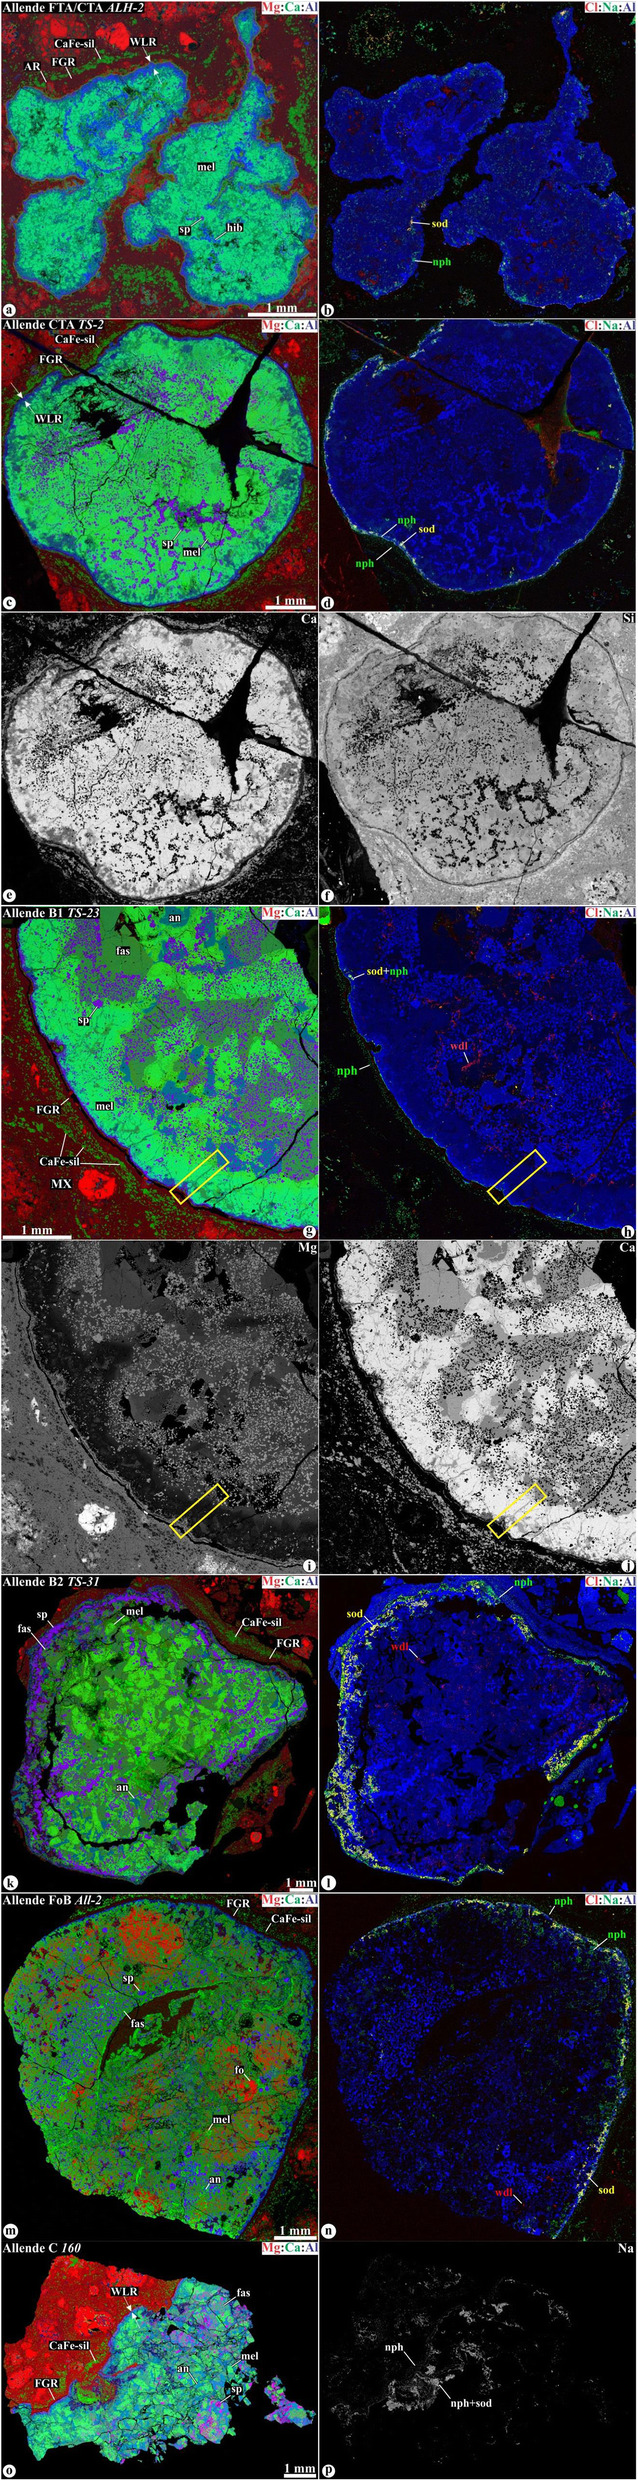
Combined x-ray elemental maps in (a, c, g, k, m, o) Mg (red), Ca (green), and Al (blue), (b, d, h, l, n) Cl (red), Na (green), and Al (blue) and elemental maps in (e, j) Ca, (f) Si, (j) Mg, and (p) Na of representative samples of coarse-grained CAIs from the CV3 carbonaceous chondrites Allende studied: *ALH2* (FTA/CTA), *TS2* (CTA), *TS23* (B1), *TS31* (B2), *Al-2* (FoB), and *160* (C). Regions outlined in “g−j” are shown in detail in Fig. X. All CAIs are surrounded by Wark-Lovering rims (WLR), fine-grained matrix-like rims (FGR), and a layer of Ca,Fe-rich silicates (CaFe-sil). Nepheline and sodalite are concentrated in the peripheral portions of CAIs. Abundant nepheline grains occur also in fine-grained matrix-like rims. Wadalite/adrianite are found exclusively in cores of Type B CAIs (high Cl signal in b comes from epoxy). fas = Al,Ti-diopside; mel = melilite. Hereafter: abbreviations of secondary minerals are listed in Table 1
